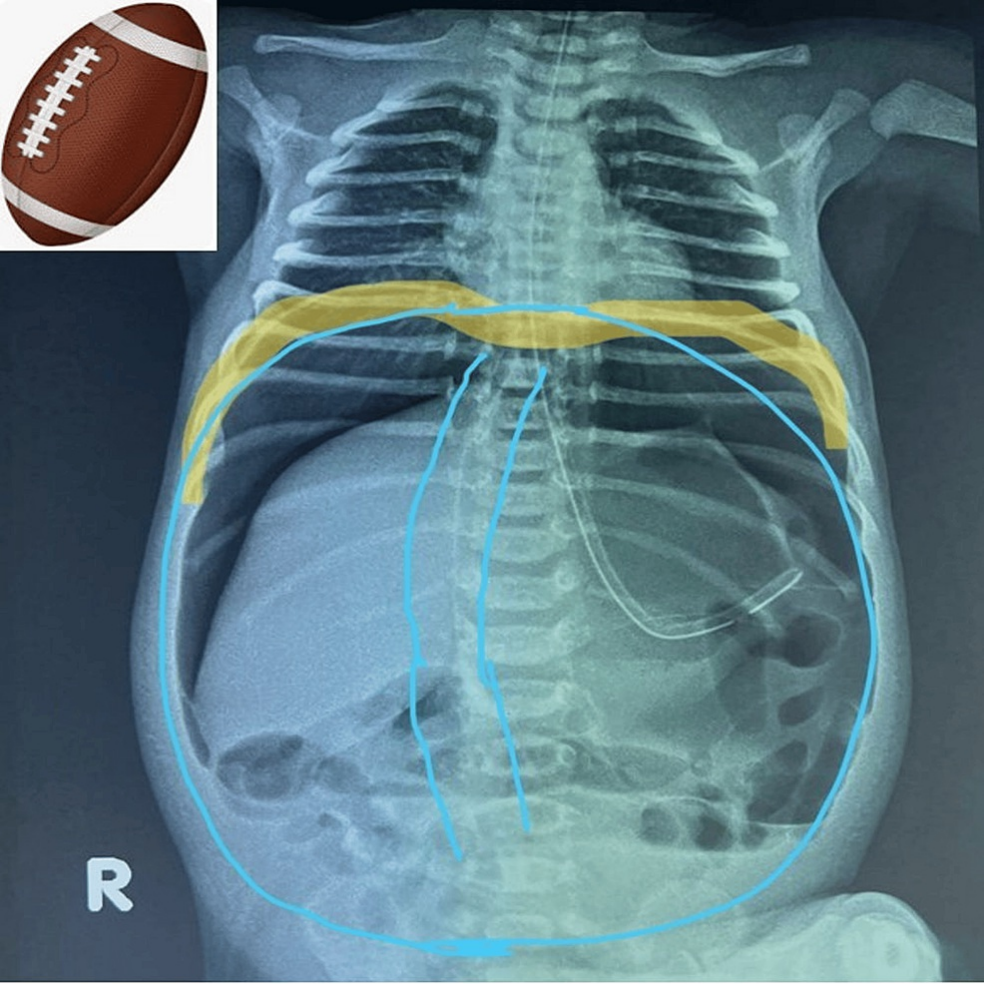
Erect abdominal X-ray of case 1 demonstrating “continuous diaphragm sign” and “football sign”
